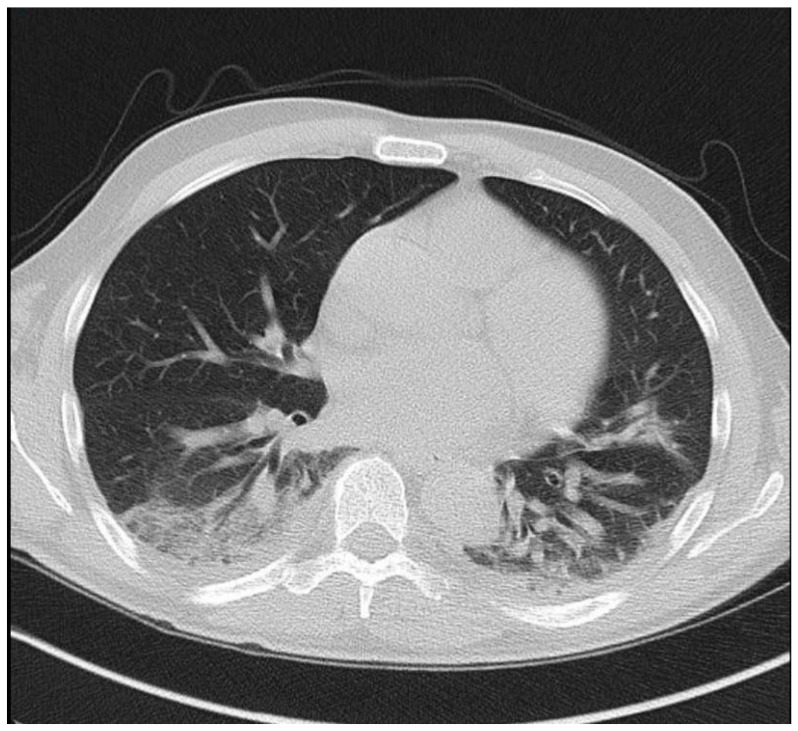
Multifocal ground glass density lesions were observed on a CT scan in the lower lobes bilaterally.
